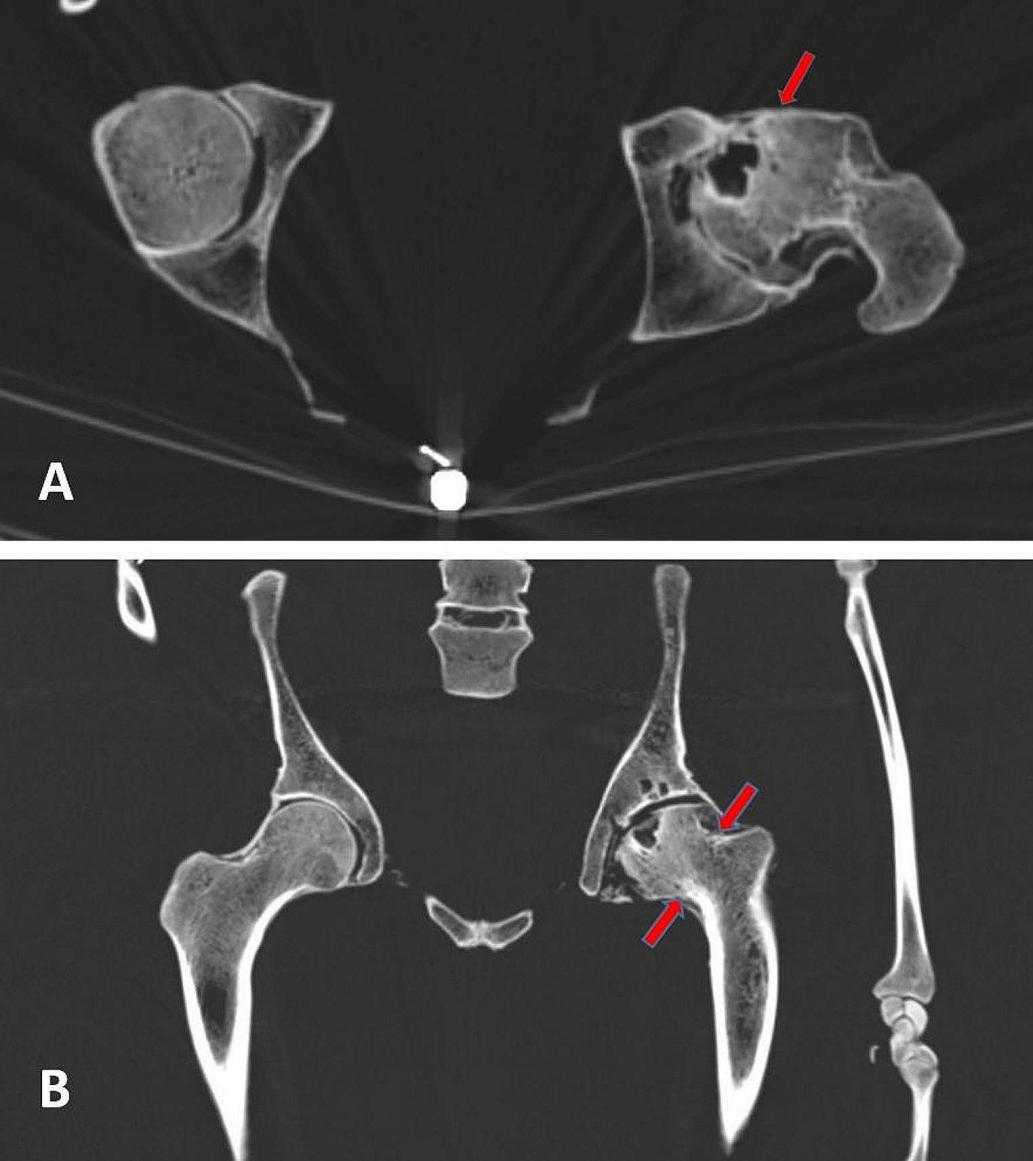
CT-scan of the left hip joint with severe posttraumatic arthrosis. (A) Horizontal section through both hip joints shows severe osteoarthrosis of the left hip joint. (B) On a coronal section the impaction of the femoral head strongly suggests an old-healed fracture (between the red arrows). This coronal section also demonstrates the coxa vara position of the left femoral head
